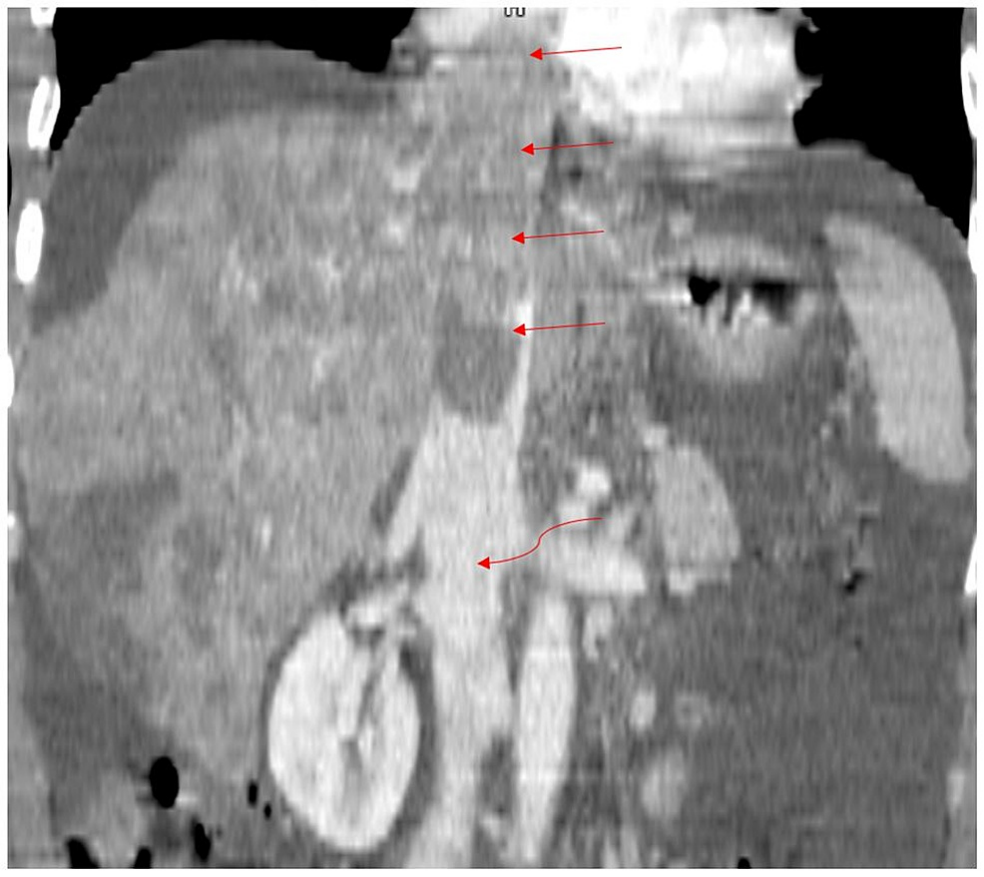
Coronal reformatted post-contrast CT demonstrating extensive tumor with invasion into hepatic venous drainage.
